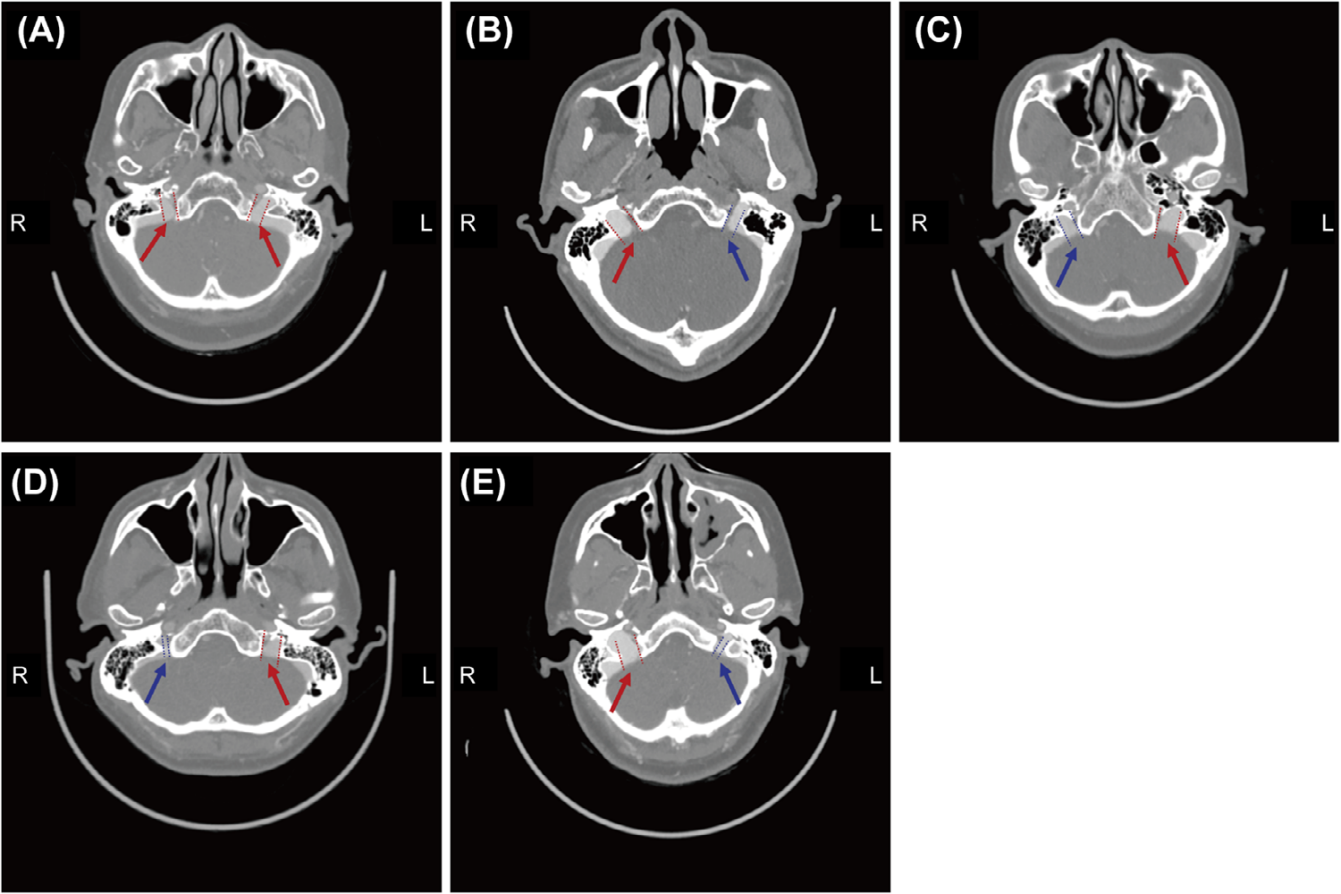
Modalities of the internal jugular foramen on CT (red and blue arrows): (A) balanced type, (B) right dominant type, (C) left dominant type, (D) right dysplastic type, and (E) left dysplastic type.
